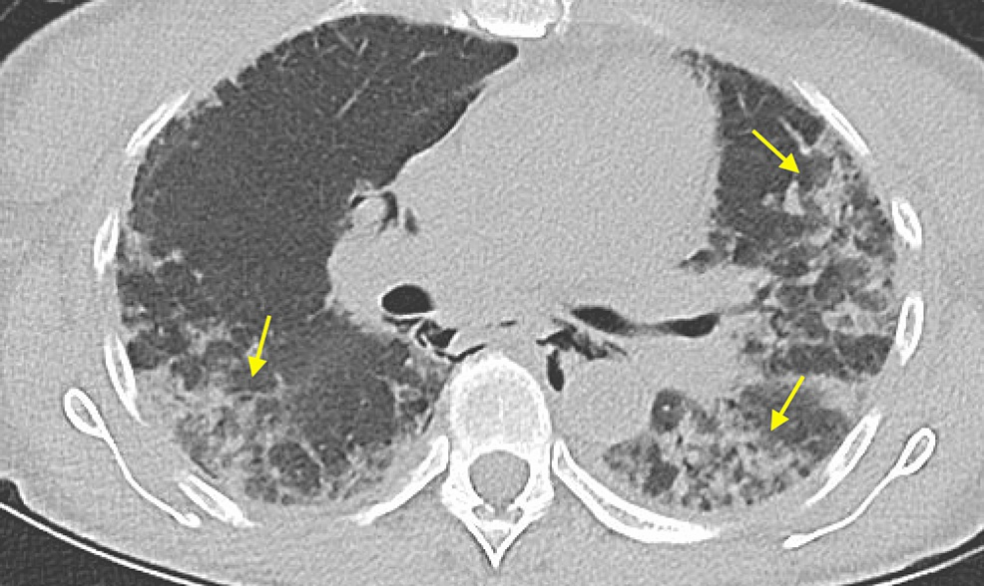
Axial view of CT chest displaying bilateral ground glass opacities and consolidation (yellow arrows)
